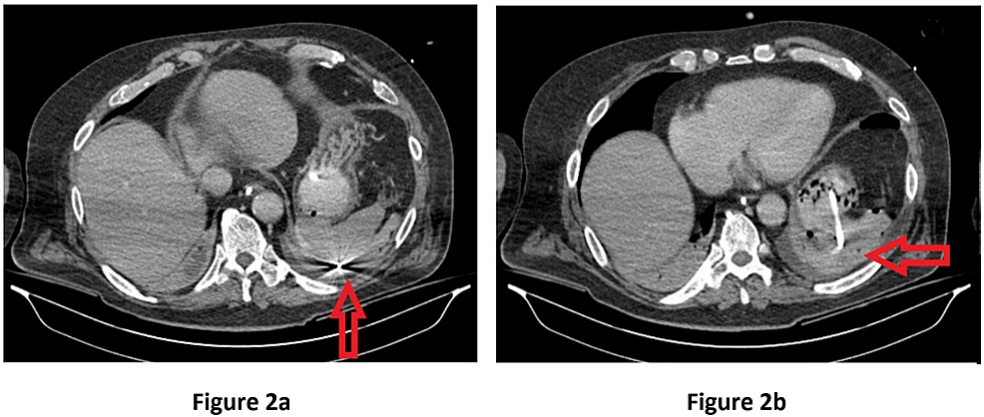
CT imaging showing a misplaced tube behind the spleen (2a) and a perisplenic collection (2b).
